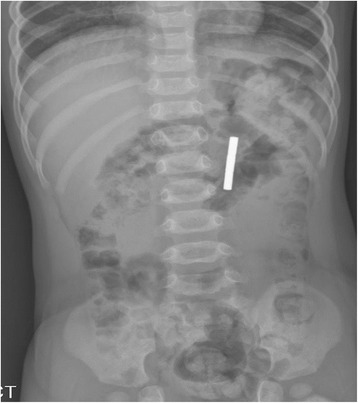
Abdomen radiography showed rod shaped metallic foreign body on left upper quadrant
